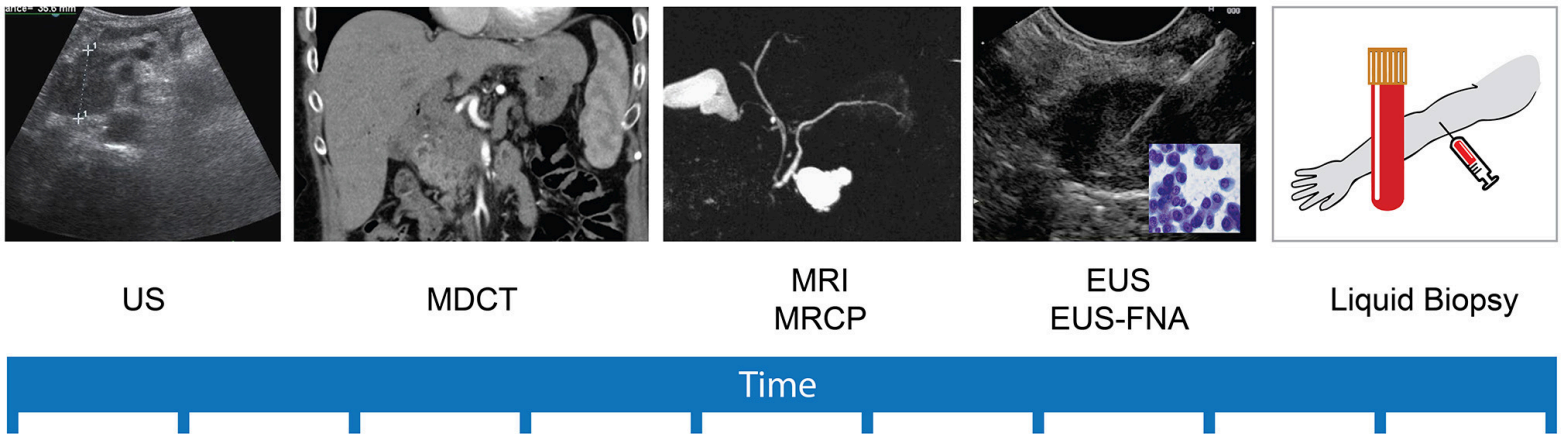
Timeline of development of pancreatic cancer diagnostic and staging modalities. Pancreatic cancer (PC) diagnosis and staging depends, substantially, on imaging modalities. Abdominal Ultrasonography (US) was the first to appear, but lacks sensitivity to detect small treatable lesions. Multidetector computed tomography (MDCT) is nowadays frequently used to detect and stage pancreatic masses, with good accuracy specially for 2 cm and larger lesions. Magnetic resonance imaging (MRI) and its variant magnetic resonance cholangiopancreatography (MRCP) improved the sensitivity for characterization small cystic lesions. More recently, endoscopic ultrasonography (EUS) with the possibility to perform fine needle aspiration (FNA) constitutes a prime modality for precise diagnosis and local staging of small (< 2 cm) solid and cystic pancreatic lesions. The innovative concept of liquid biopsy refers to a simple and painless collection of a body fluid sample (usually blood), in order to study proved and anticipated biomarkers with the potential to detect PC in its early non detectable stages and even the premalignant precursors.
